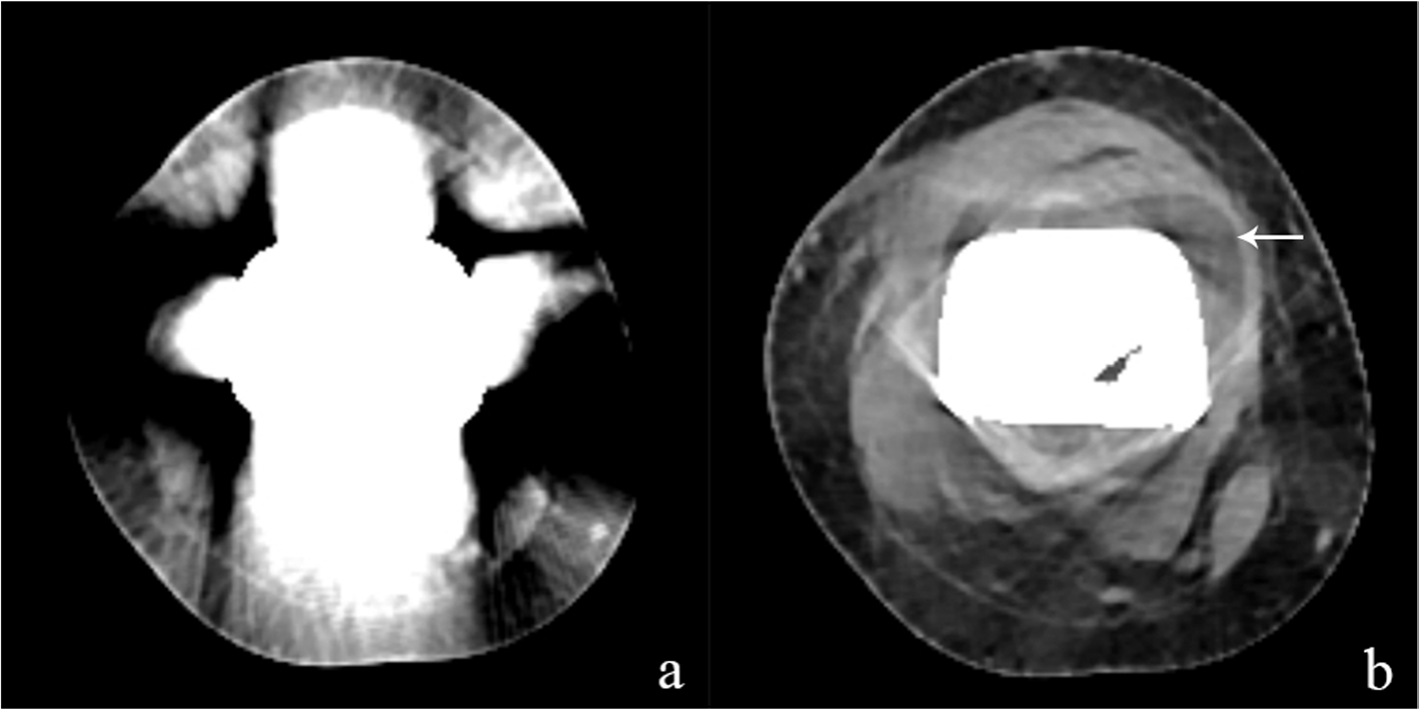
The unenhanced CT images of a 22-year-old woman with a distal femur tumor prosthesis show periprosthetic structures were almost obscured by metal artifacts on the IR image (a), which are visibly eliminated on SEMAR image, and the periprosthetic effusion (arrow) appears
